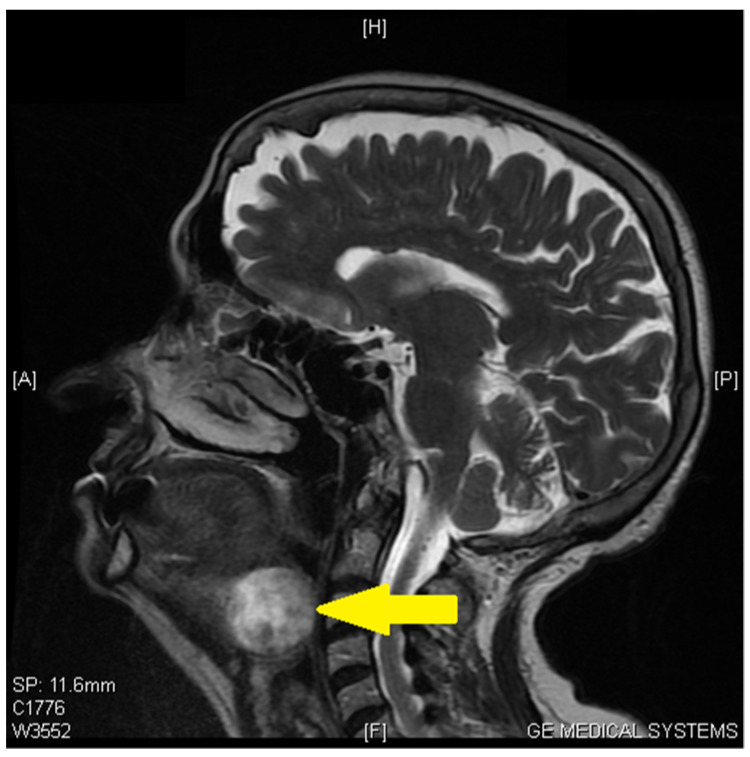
Magnetic Resonance Imaging (MRI) imaging shows a mass in the sublingual region (arrow).
